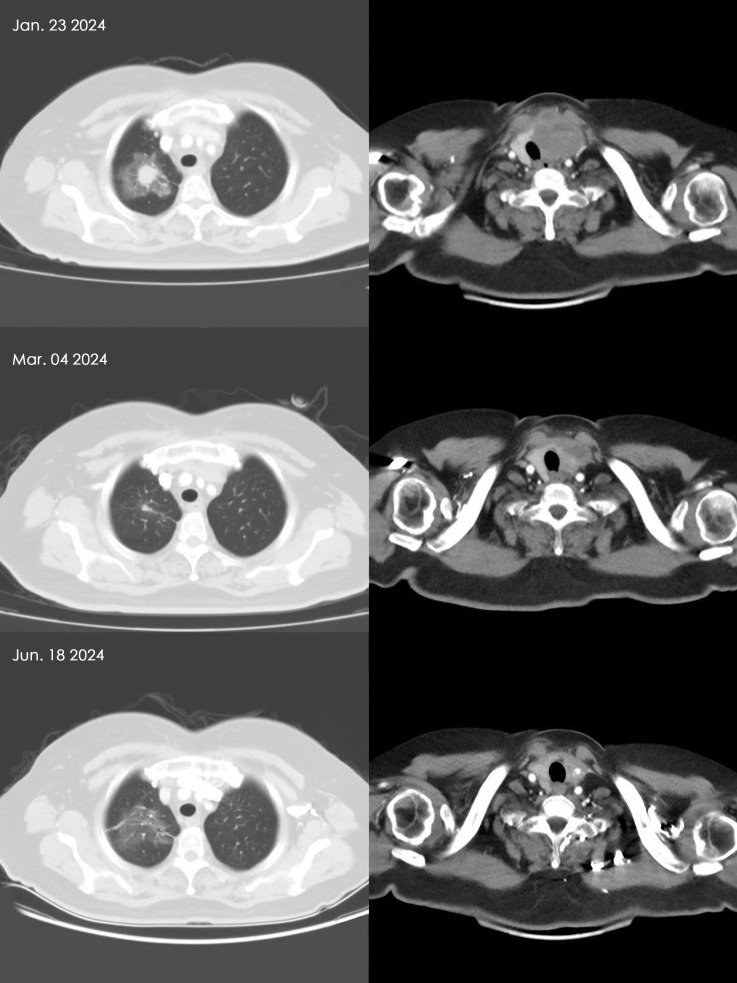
Comparison of thyroid and lung metastatic nodules in Patient D before and after combined therapy. Based on the patient’s CT scan findings, it is evident that the thyroid carcinoma and pulmonary metastatic lesions have appreciably regressed following 6 weeks and 21 weeks of combination therapy.
